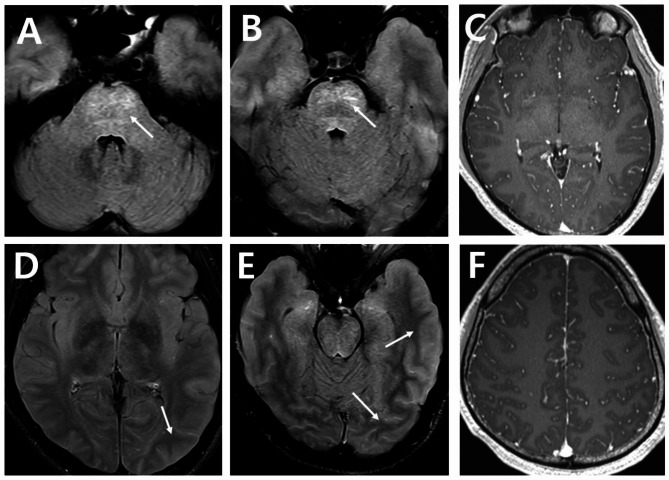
Initial brain magnetic resonance imaging with gadolinium-enhancement. (A) (B) FLAIR imaging with gadolinium-enhancement revealed subtle high signal intensity in the central pons suggestive of meningoencephalitis (D) (E) FLAIR imaging with gadolinium-enhancement revealed diffuse leptomeningeal enhancement combined with diffuse cortical swelling and crowding gyri suggestive of increased intracranial pressure and meningoencephalitis (C) (F) T1-weighted gadolinium-enhancement revealed diffuse leptomeningeal enhancement
